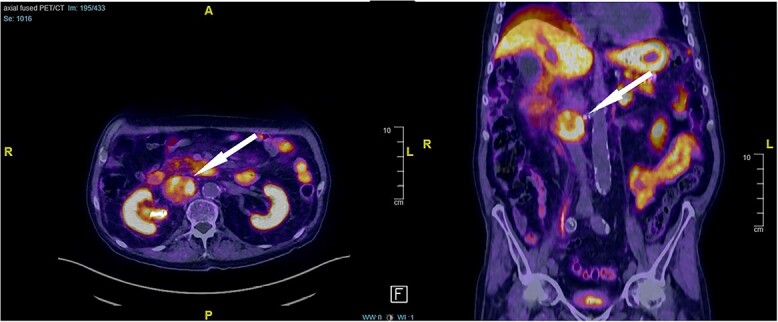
DOTATE-PET/CT: sizeable retrocaval mass with edge-accentuated radionuclide storage, compatible with a paraganglioma or a lymph node metastasis of a neuroendocrine tumor (arrow).
